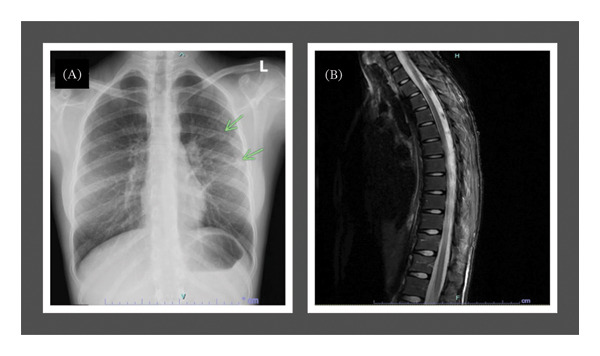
(A) X‐ray chest PA and lateral done on the day of admission showing patchy left upper lobe infiltrate; no pleural effusion or pneumothorax noted. (B) MRI thoracic spine with or without contrast performed on HD 8 demonstrating the enhancement of both the ventral and caudal nerve roots of the mid to lower thoracic spine. There also appears to be enhancement of the cauda equina nerve roots. No lumbar spine MRI was performed.
